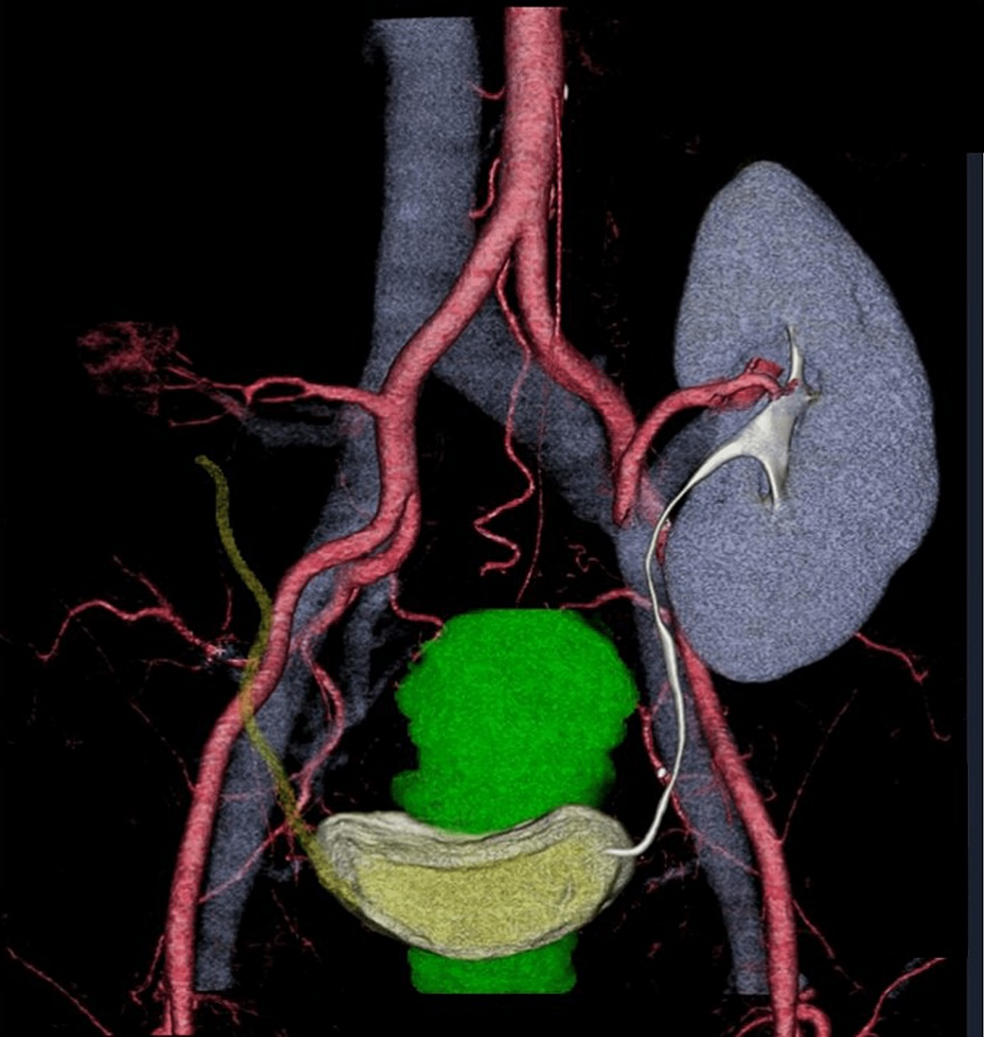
Three-dimensional computed tomography Three-dimensional computed tomography reveals the relationship between the arteriovenous vessels of the transplanted left kidney and left iliac vessels and the left ureter of the transplanted left kidney.
